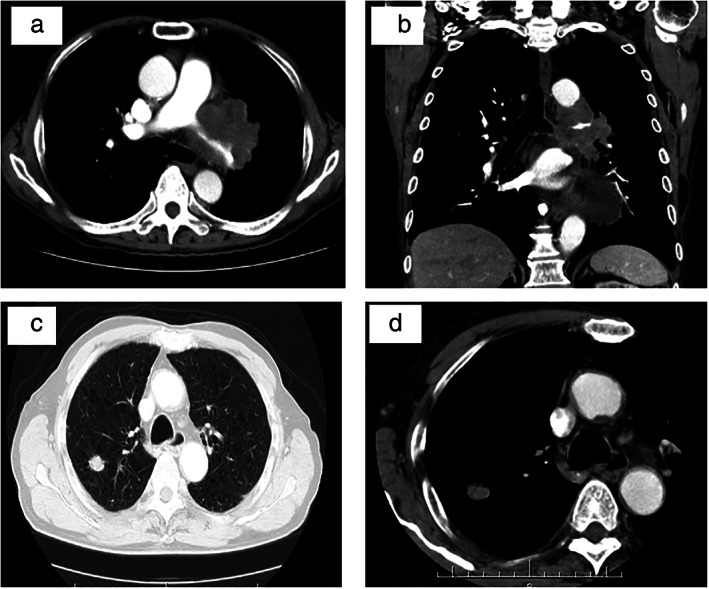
Representative images of the tumor extension and spread pattern of central and peripheral types of small‐cell lung cancer. Typical thin‐sliced computed tomography images of the central (a and b) and peripheral (c and d) types
